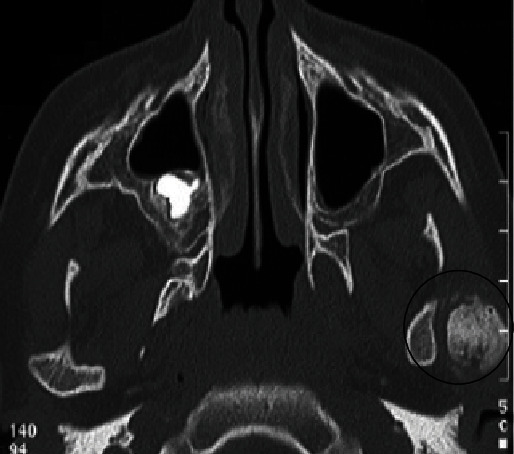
Axial computed tomography slice in bone rendering showing ankylotic mass (black circle) located laterally to the left condyle.
